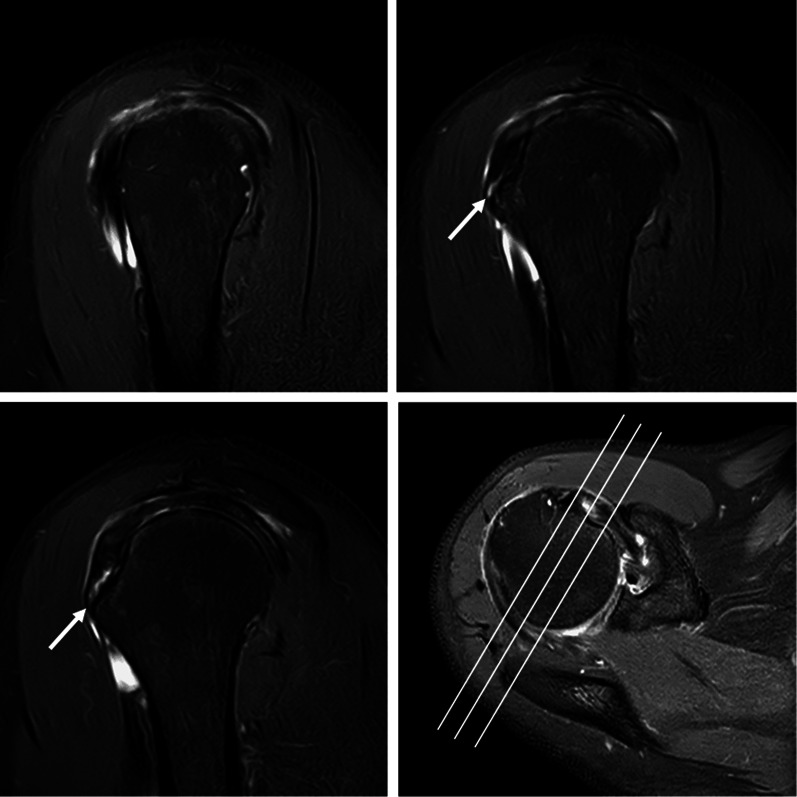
Type-A of fissure sign. There is a transverse fluid-filled gap in the interface between cortex and tendon of SSC footprint at the first three slices on oblique sagittal IM-WI/fs MRI which counted from the most lateral cortex of lesser tuberosity to the medial SSC footprint area referring to the axial MR image, and must be found at least two continuous slices (white arrow)
